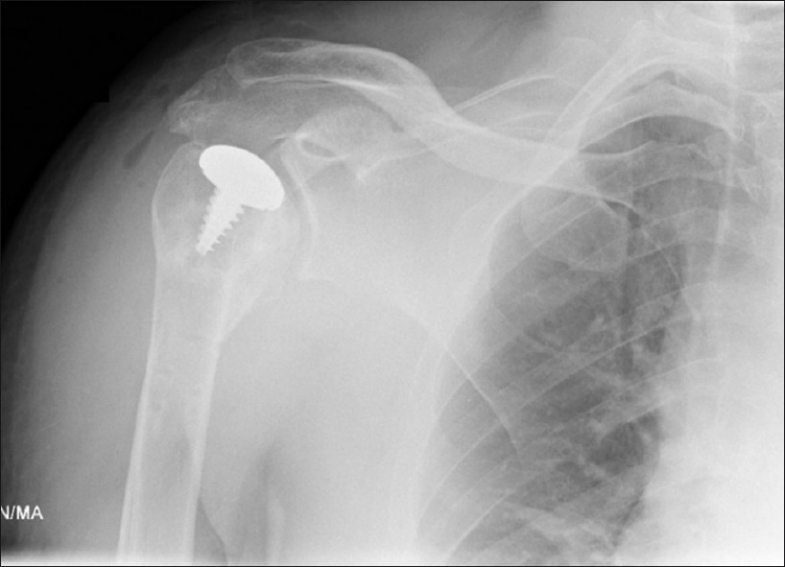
Check radiograph postoperatively
